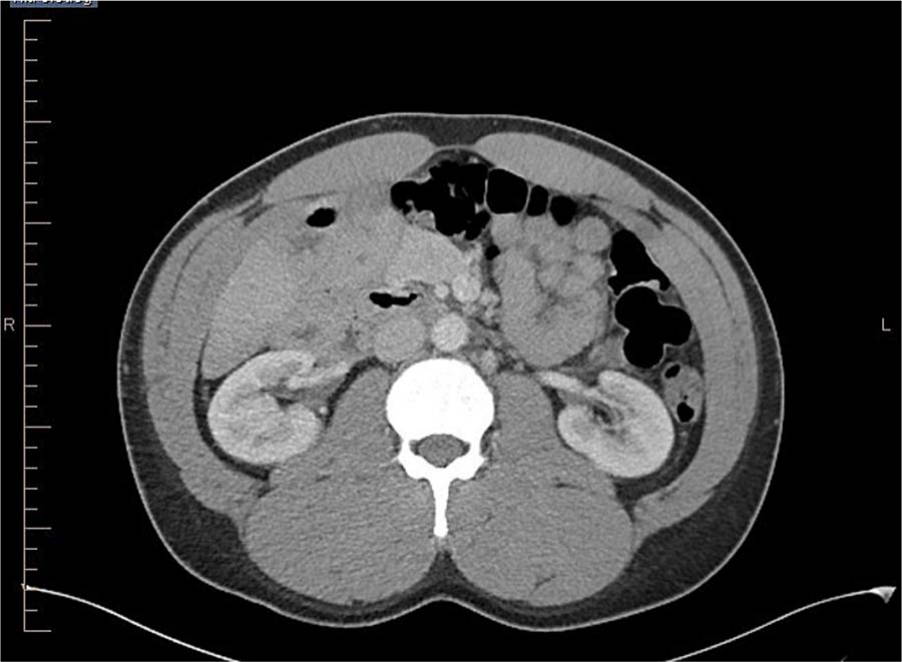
Axial view of abdominal CT scan with contrast showing the reversal of SMA and SMV.
